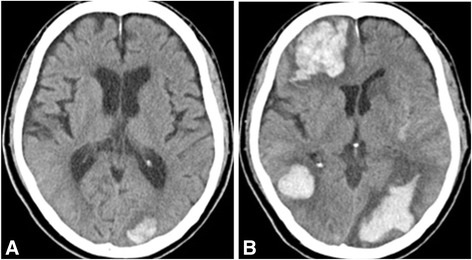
Head computed tomography (CT) scans after thrombolysis. A: CT scan right after the neurological deterioration showing a subcortical hemorrhage in the left occipital lobe. B: Repeat CT scan the day after onset showing enlargement of the left occipital hemorrhage and two subcortical hemorrhages in the right frontal and right temporal lobes.
